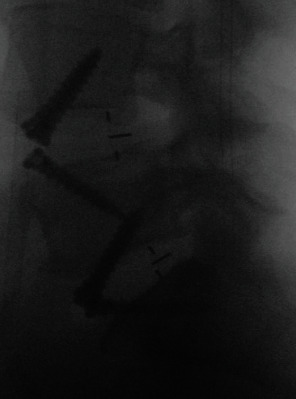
Intraoperative sagittal X-ray demonstrating anterior interbody grafts.
